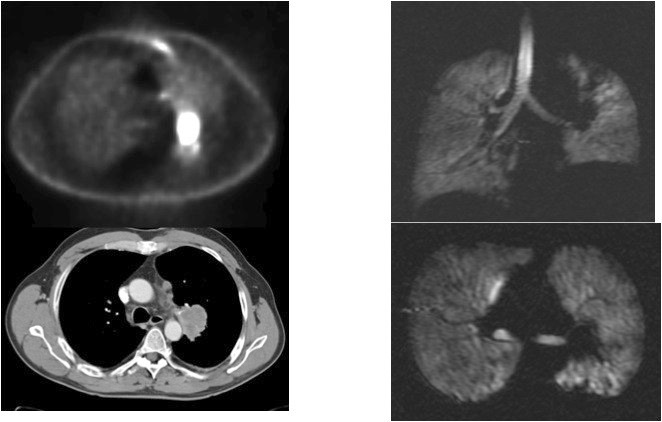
Patient #3 – with central partially obstructing mass on CT, PET and Helium MRI.
